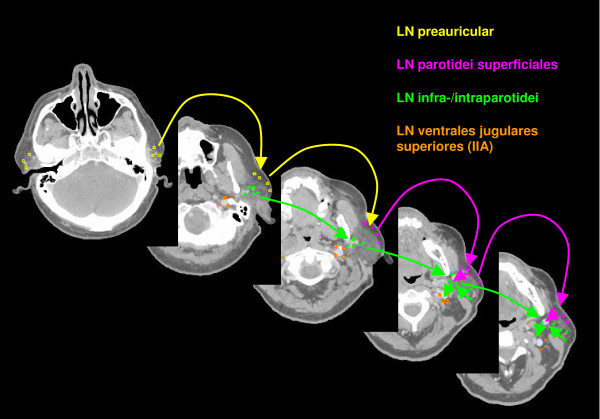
Lymph regions and drain contoured in transversal CT slices: LN parotidei superficiales (pink) and LN parotidei profundi subdivided into LN preauriculares (yellow) and LN infra-/intraparotidei (light green) [1.8 cm slice thickness].
